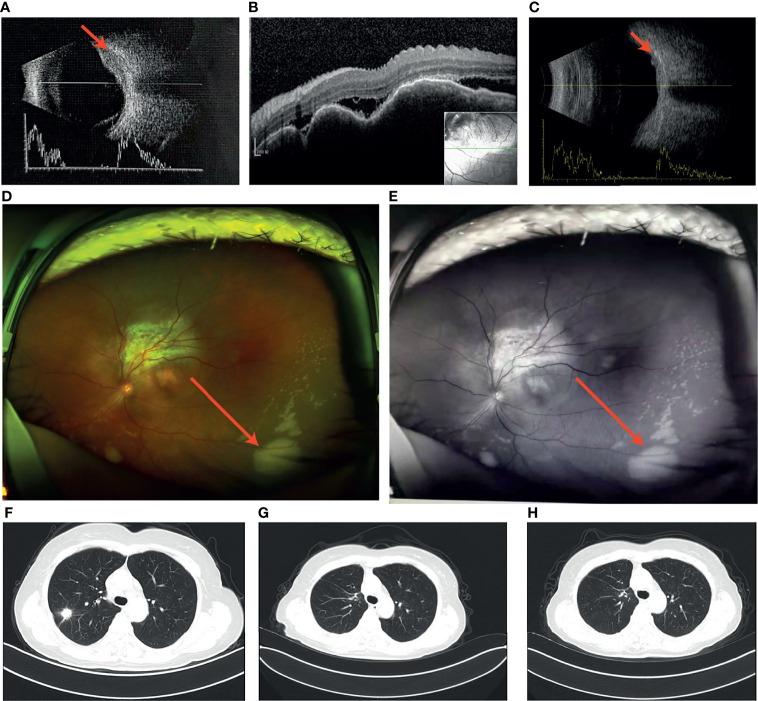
(A) Ultrasound revealed the initial dome-like choroid lesion with the height 2.5 mm: (B) Optical coherence tomography (OCT) demonstrated a plateau-like elevation of choroid with underlying sub retinal fluid; (C) The height of the lesion reduced to 0.75 mm 18 months after crizotinib therapy; (D,E) Left fundus image revealed a large dome-shaped amelanotic tumor; (F) A nodule in the right upper lung; (G) Regression of the primary lesion 2 month after surgery; (H) No progression of malignancy in the 18th month of crizotinib therapy.
